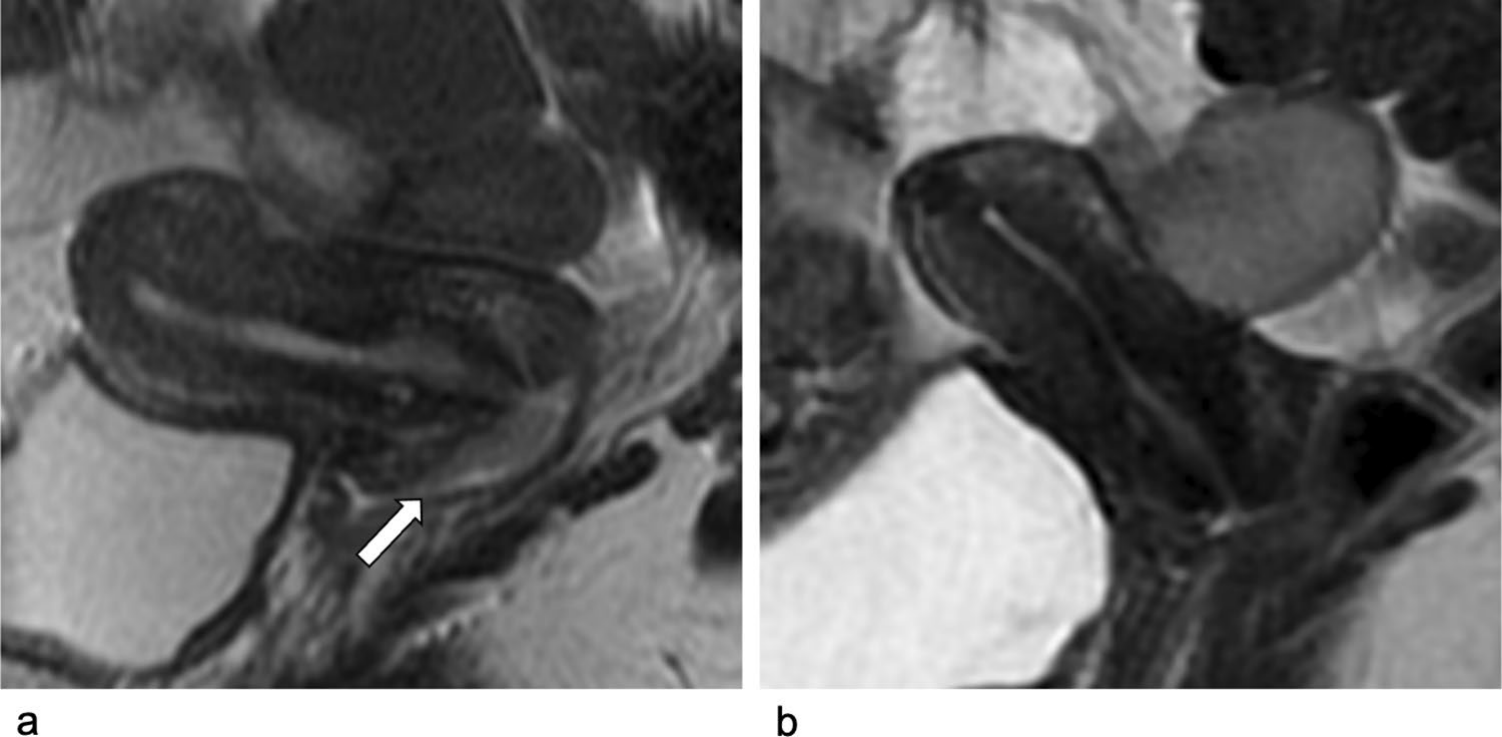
Baseline and post-NACT MRI in 34-year-old woman with cervical adenocarcinoma (FIGO IB2). a, b Sagittal T2-w images. At baseline MRI (a), sagittal T2-w images show the hyperintense cervical mass (arrow), with maximum diameter of 25 mm. After NACT (b), T2-w sequences show no evidense of residual disease both on T2-w images (b, c) and on DWI images (d), configuring a CR to NACT. NACT: neoadjuvant chemotherapy; CR: complete response
